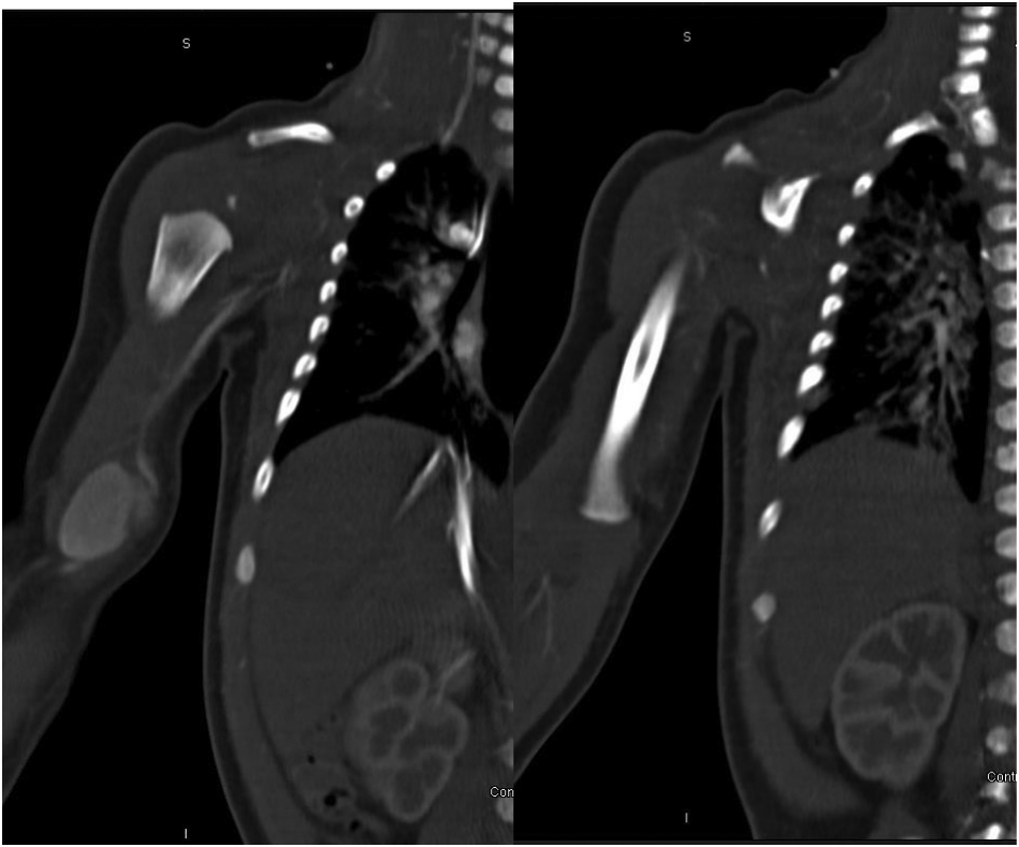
Contrast-enhanced magnetic resonance image showing a mass containing contrast at the medial aspect of the right brachial artery measuring 1.6 × 1.8 cm in cross section and 2.1 cm in craniocaudal dimension. Contrast opacification of the mass was present in the arterial phase secondary to communication with the brachial artery lumen. No solid contents were within the mass and no filling defects were present. The pseudoaneurysm extended to the subcutaneous soft tissue with no surrounding collection or edema seen.
